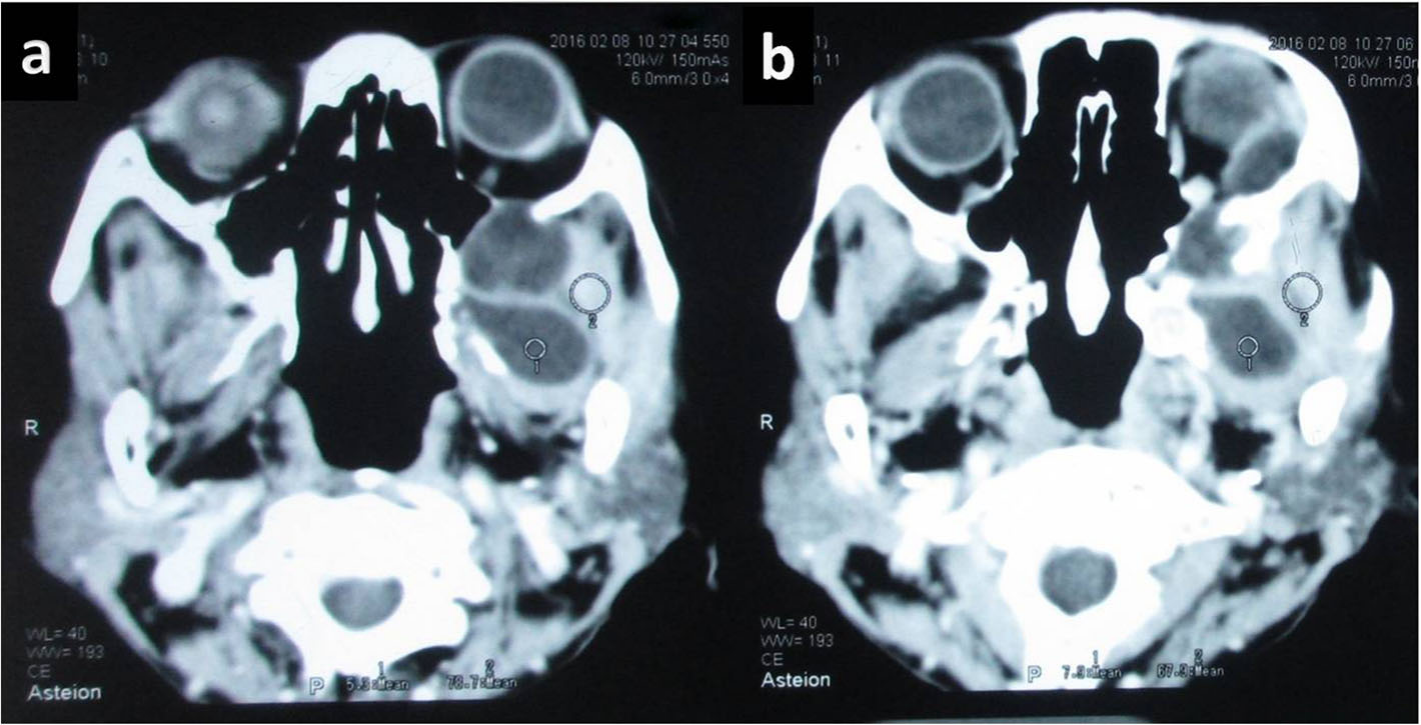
Axial computed tomography images of head and orbit showing multiloculated cystic lesion involving the left infratemporal fossa (a) extending to the extraconal space of left orbit (b)
